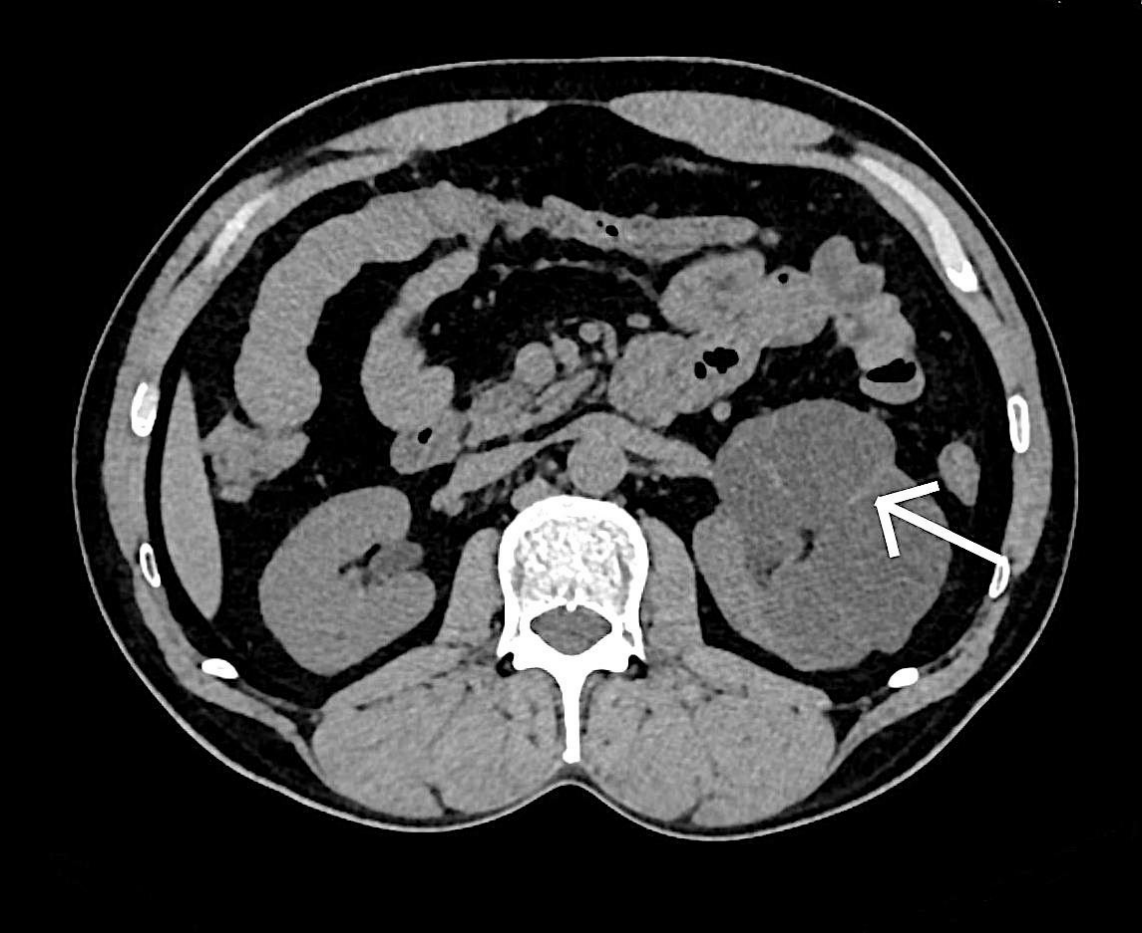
A 41-year-old female patient with complaint of left flank pain. Unenhanced axial computed tomography scan of the affected left kidney shows multiple hypodense cysts separated by thinned out residual renal parenchyma (white arrow).
